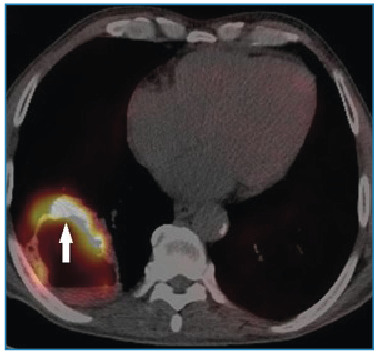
Positron emission tomography/computed tomography imaging shows hypermetabolic involvement.
